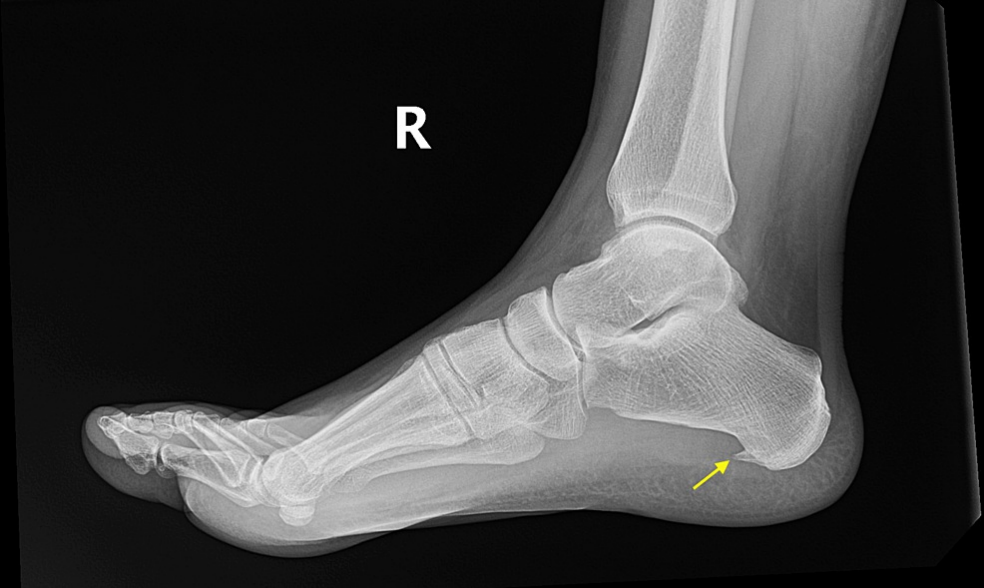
Radiograph depicting a calcaneal spur (arrow)
